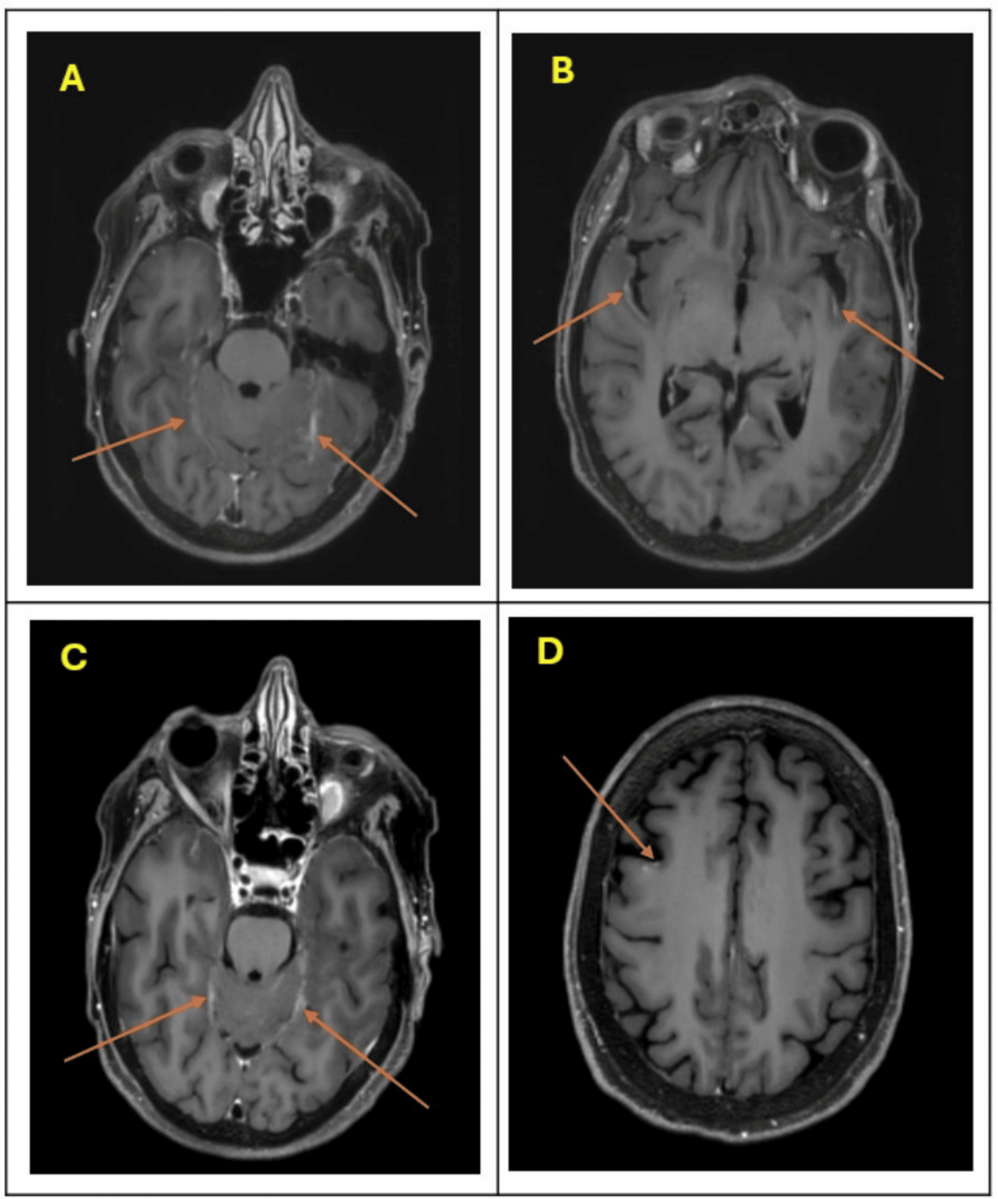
Axial contrast-enhanced brain MRI demonstrating leptomeningeal disease (LMD). Axial post-contrast T1-weighted MRI of the brain (labeled A–D) demonstrating abnormal enhancing lesions (arrows) concerning for leptomeningeal disease (LMD). (A) and (C): Enhancement along the bilateral cerebellar folia and posterior fossa leptomeninges. (B): Leptomeningeal enhancement involving the bilateral temporal lobes. (D): Focal gyral enhancement over the high convexity region, suggestive of cortical involvement. These imaging findings are characteristic of LMD, often seen in advanced metastatic disease.
